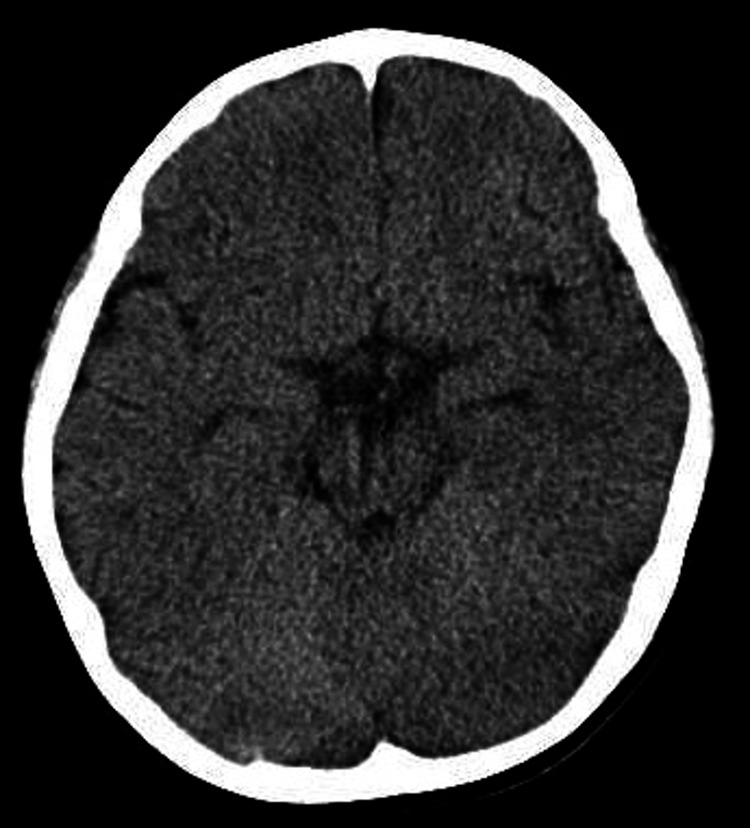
Brain computed tomography at the emergency department visit revealed no abnormality
